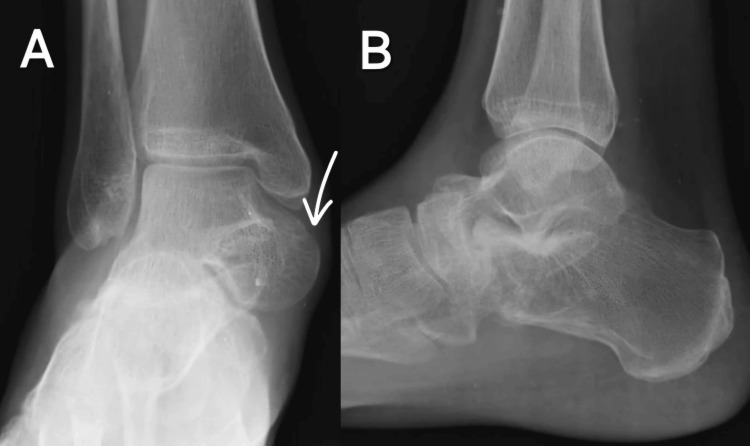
Pre-operative radiograph of the right ankle in (A) anteroposterior and (B) lateral views The white arrow shows subluxation of the talus from the ankle joint, with the navicular completely dislocated laterally, exposing the head of the talus.
